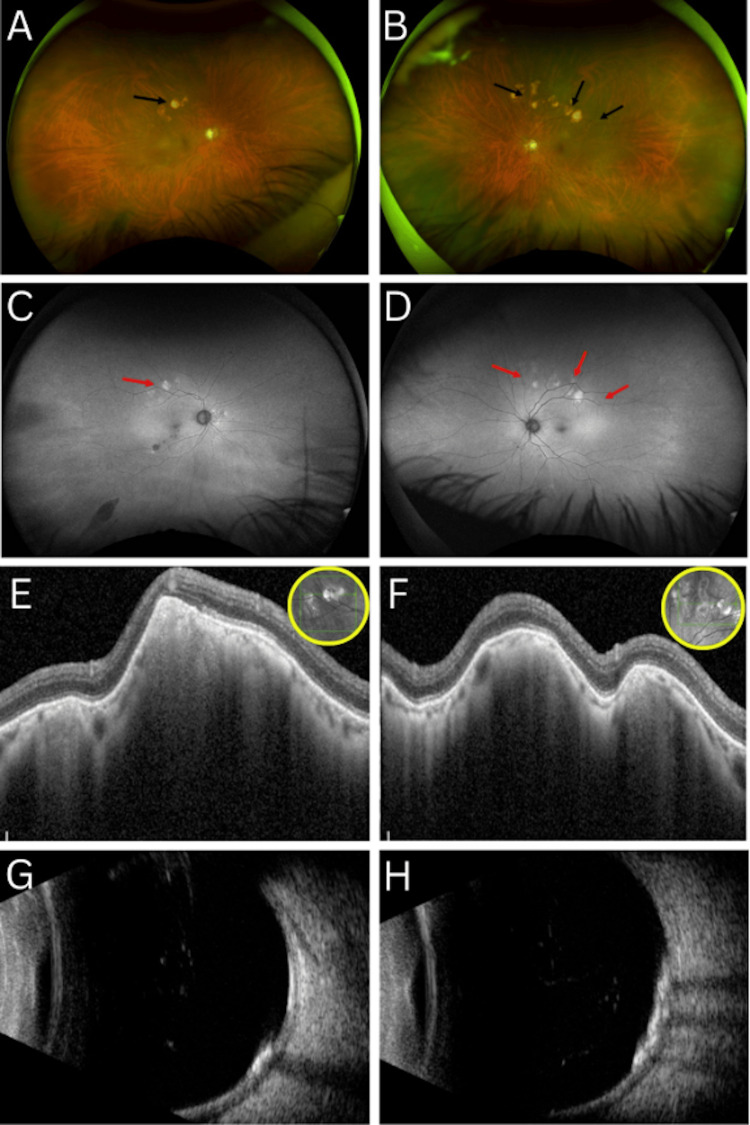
Multimodal imaging of both eyes at presentation Color fundus photographs at initial presentation of the right (A) and left (B) eyes show bilateral yellowish placoid lesions (black arrowheads) superior to the macula with associated drusen. FAF of the right (C) and left (D) eyes demonstrates mixed hyper- and hypoautofluorescent signals (red arrowheads) corresponding to the lesions. (E-F) OCT reveals choroidal elevations with increased reflectivity, while (G-H) B-scan ultrasonography confirms calcification with hyperechoic lesions and posterior acoustic shadowing in both eyes. FAF, fundus autofluorescence; OCT, optical coherence tomography
